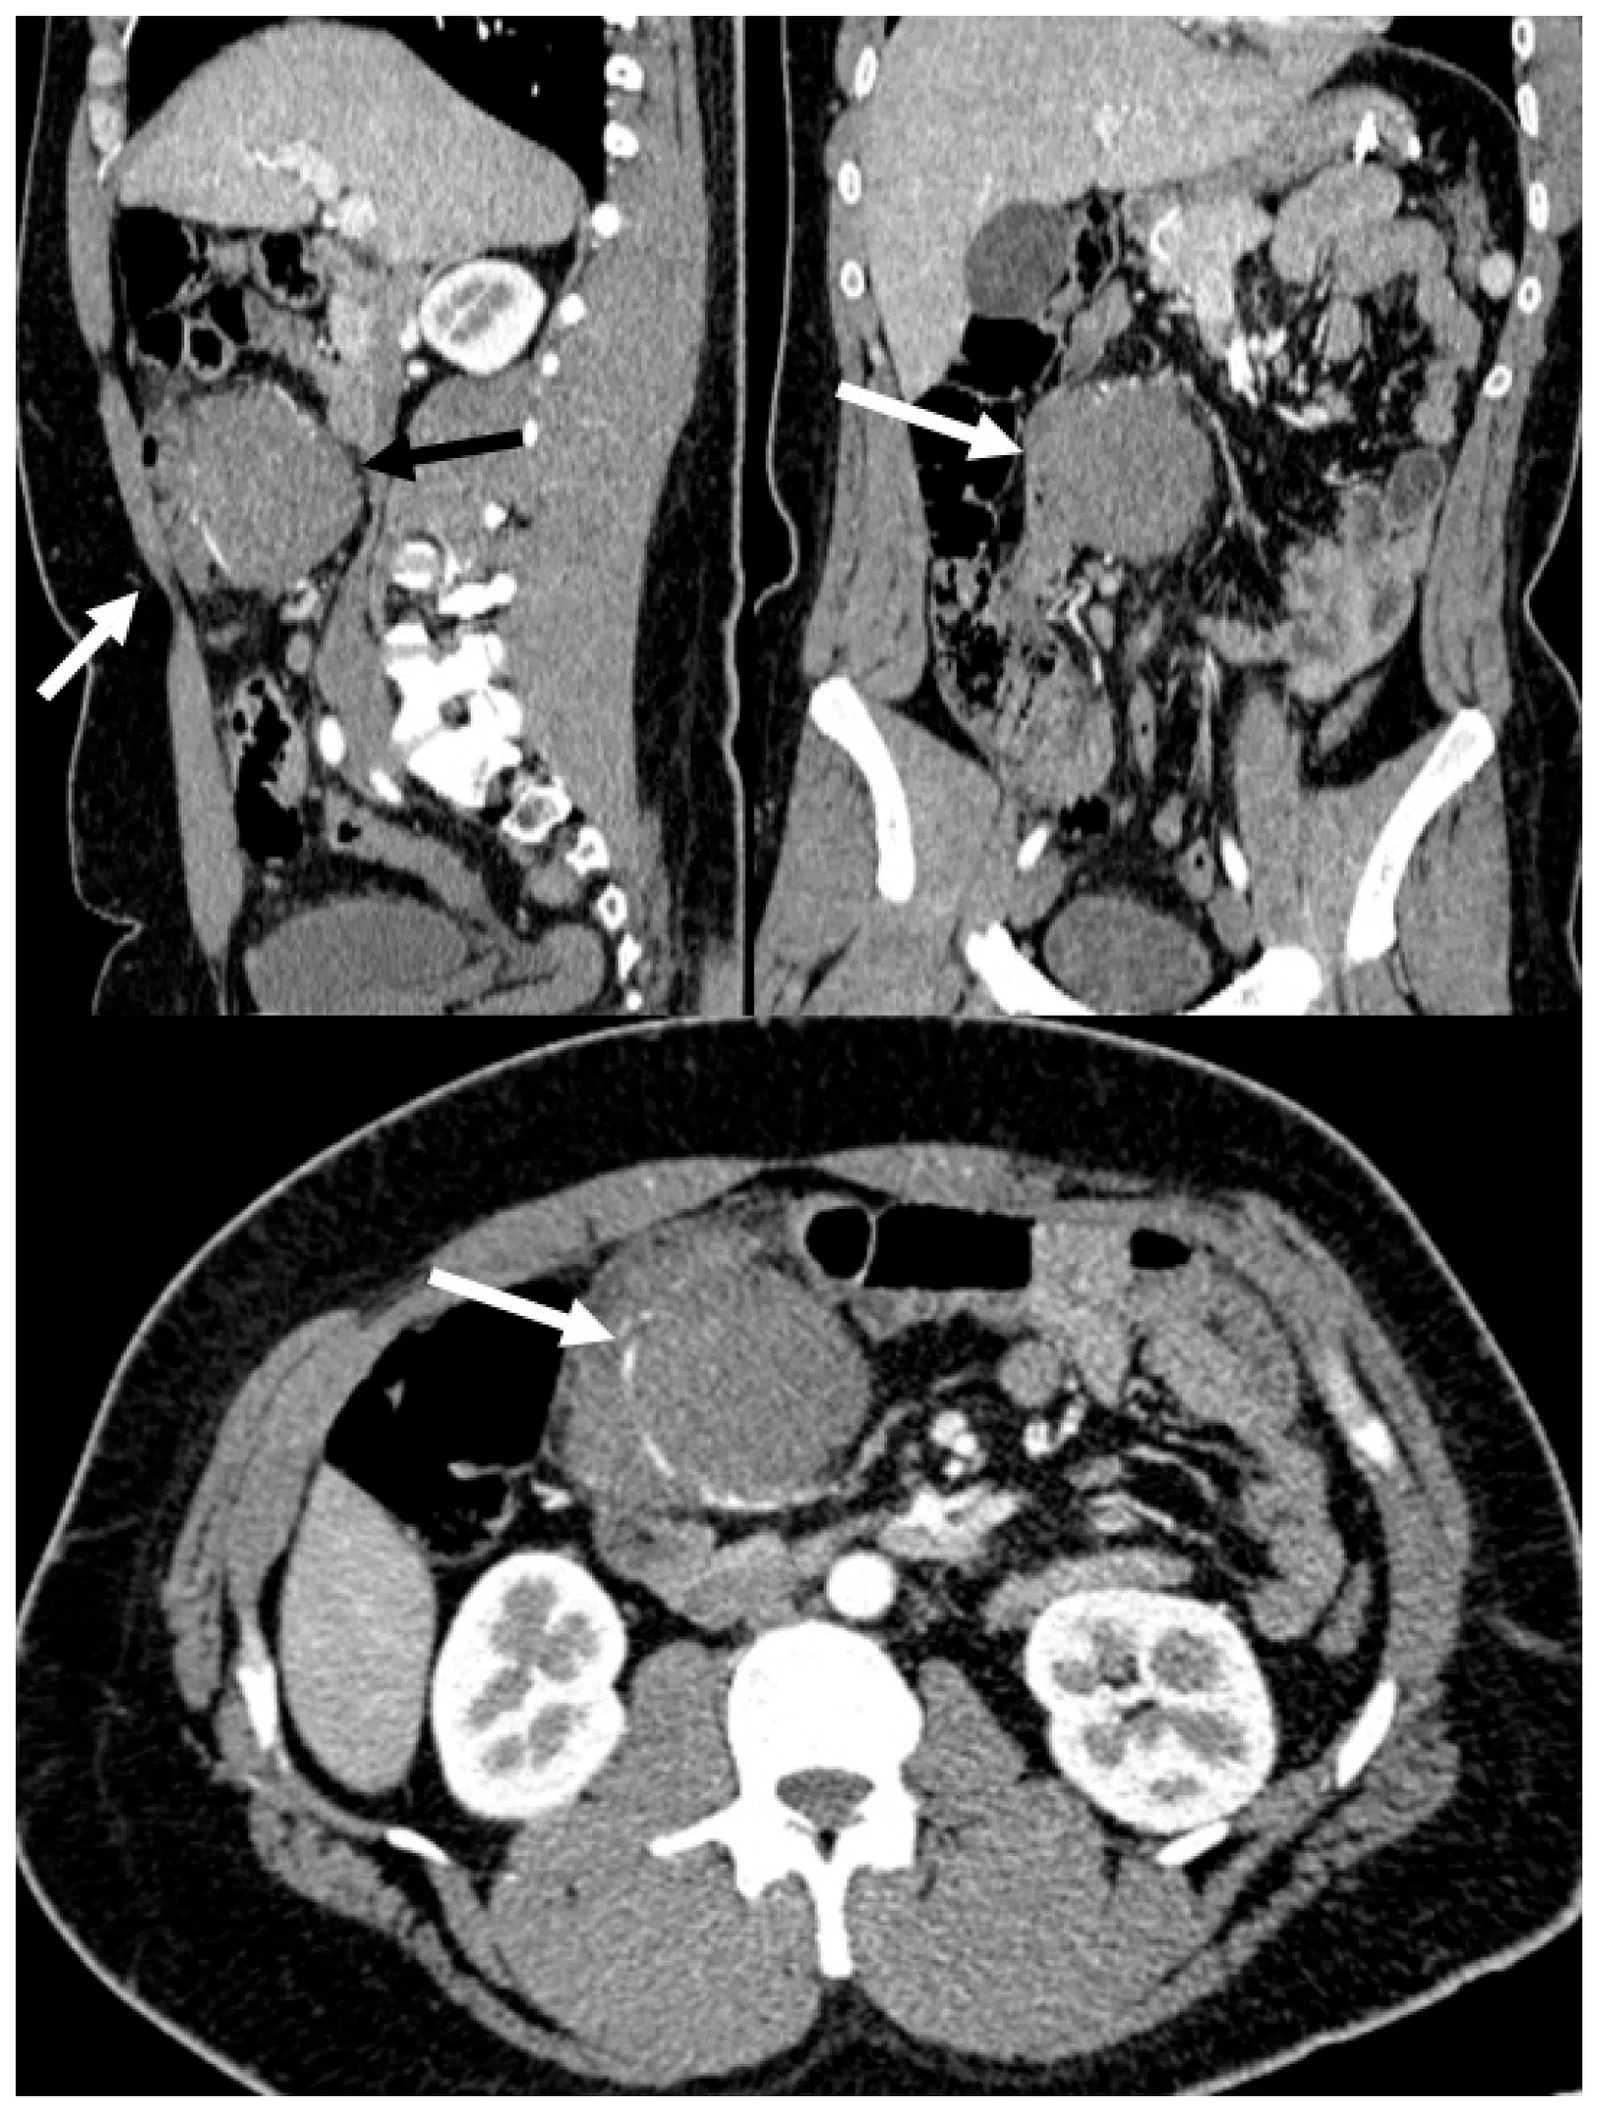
The CT scan reveals the abdominal mass (white arrows) compressing the vena cava (black arrow).
